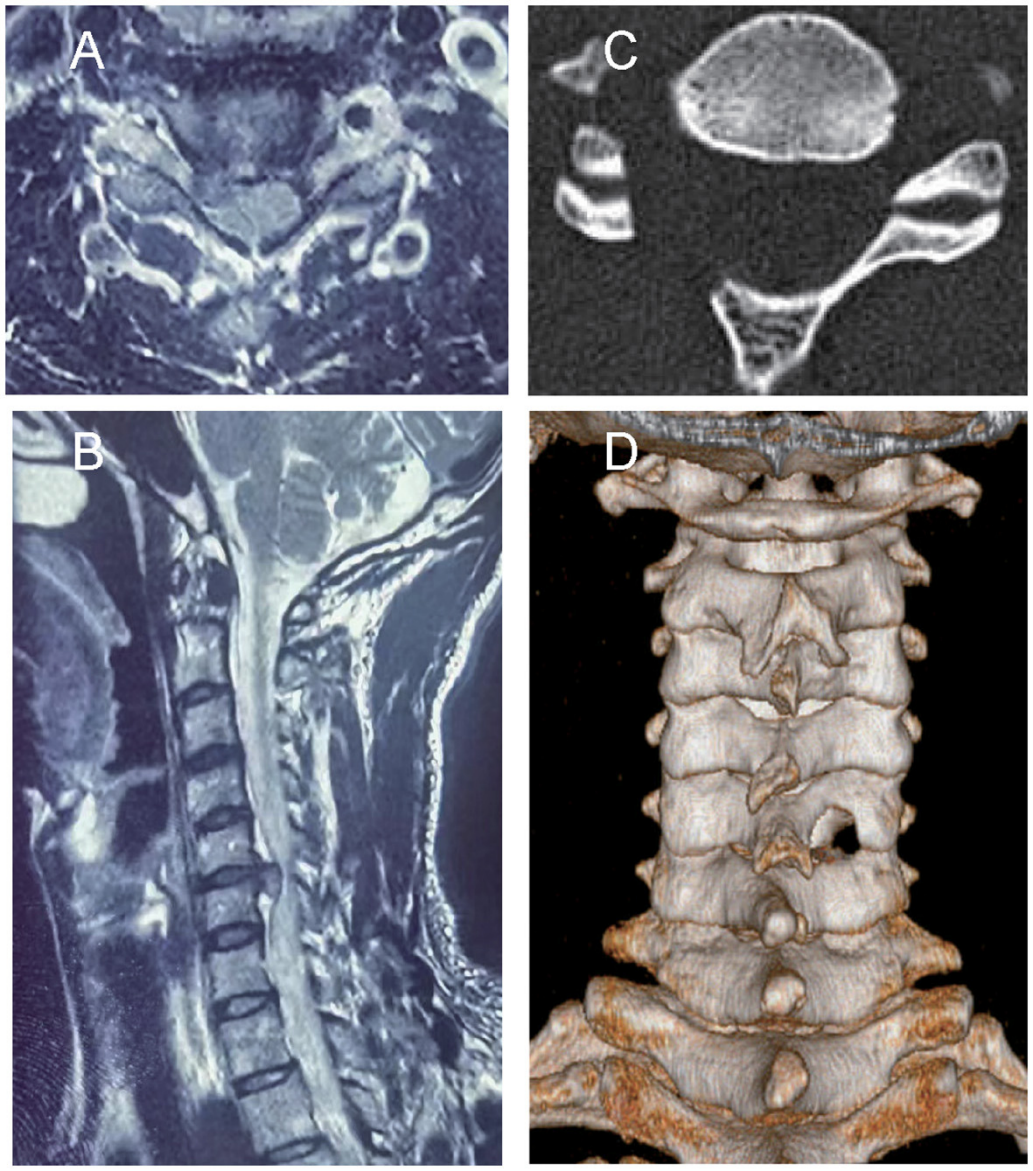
A 40-year-old man had cervical 5–6 intervertebral disc herniation, presenting with neck pain and numbness in the right upper limb. (A, B) Preoperative axial and sagittal magnetic resonance images showed C5/6 disk protrusion on the right subarticular zone. (C) Postoperative axial computed tomography scan showed the location of the unilateral foraminotomy. (D) Postoperative 3-dimensional computed tomography scan showed widening of the spinal canal after surgery.
